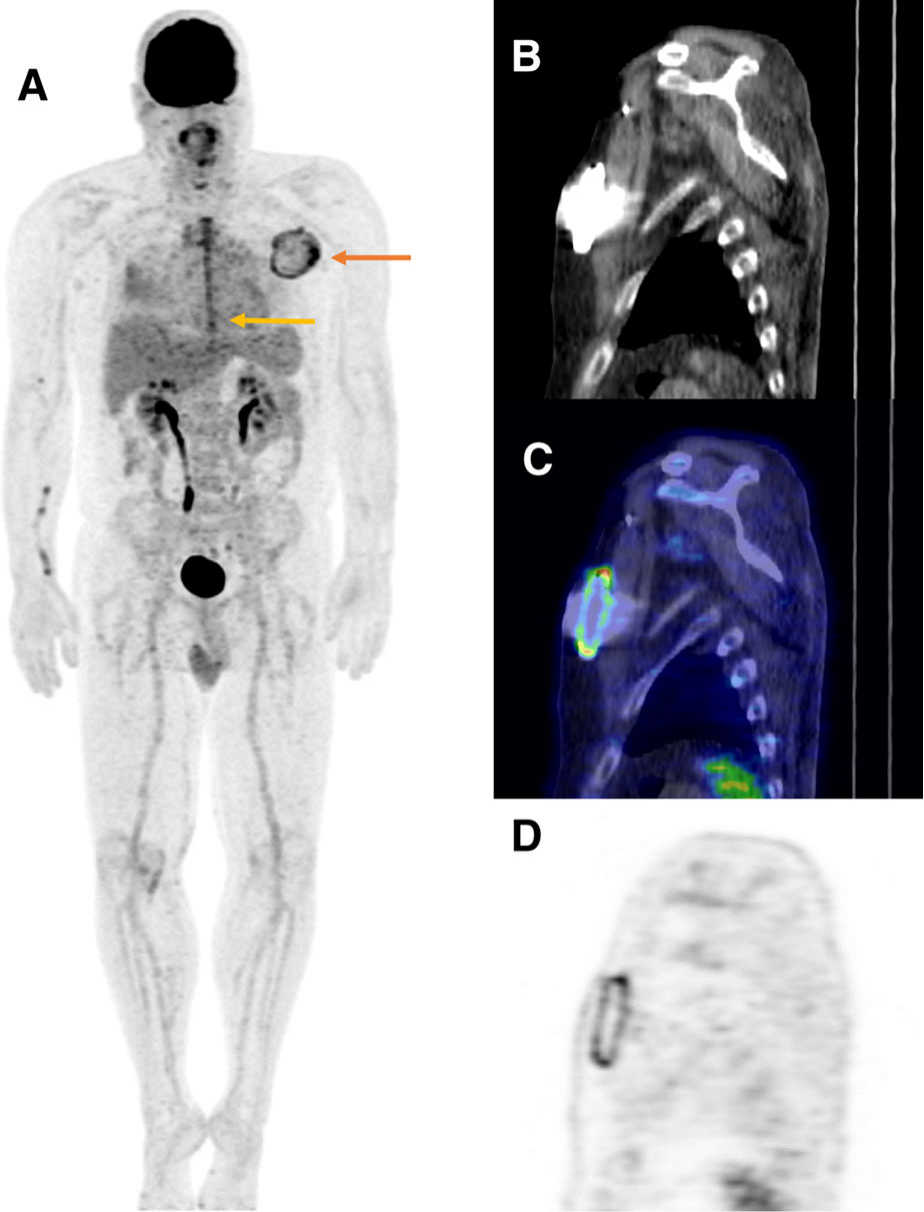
Patient #10 had CIED implanted 8 years ago and now two months after elective CABG procedure he had pain, redness and swelling in pocket area. ^18^F-FDG-PET/CT showed uptake in pocket (SUV_max_ 7.1) (Panel A, *red arrow*). There were also physiological uptake in sternotomy wound (SUV_max_ 5.3) (Panel A, *yellow arrow*). The CIED system was removed and infection was confirmed as bacterial culture which yielded *Staphylococcus epidermidis.* Panel B sagittal CT scan, Panel C sagittal fusion, Panel D sagittal PET
